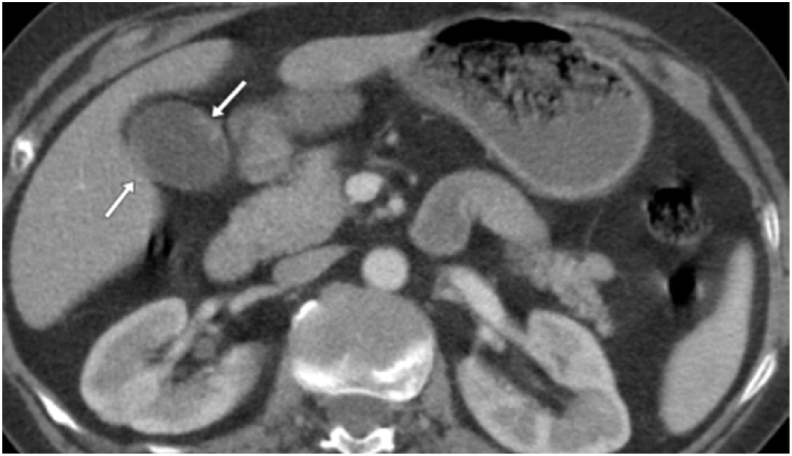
Arterial sequence of the CT scan.
